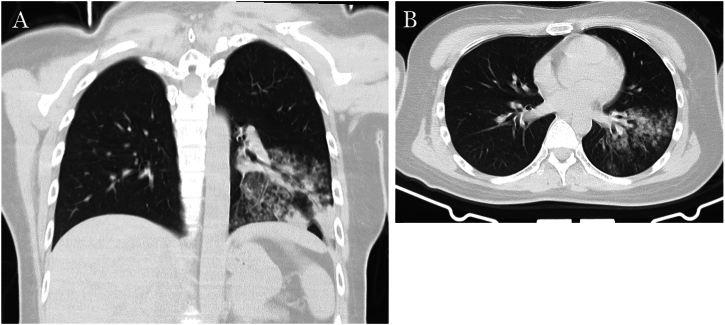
Chest CT on admission showed lung pneumonia in the left inferior lobe (A, B).
